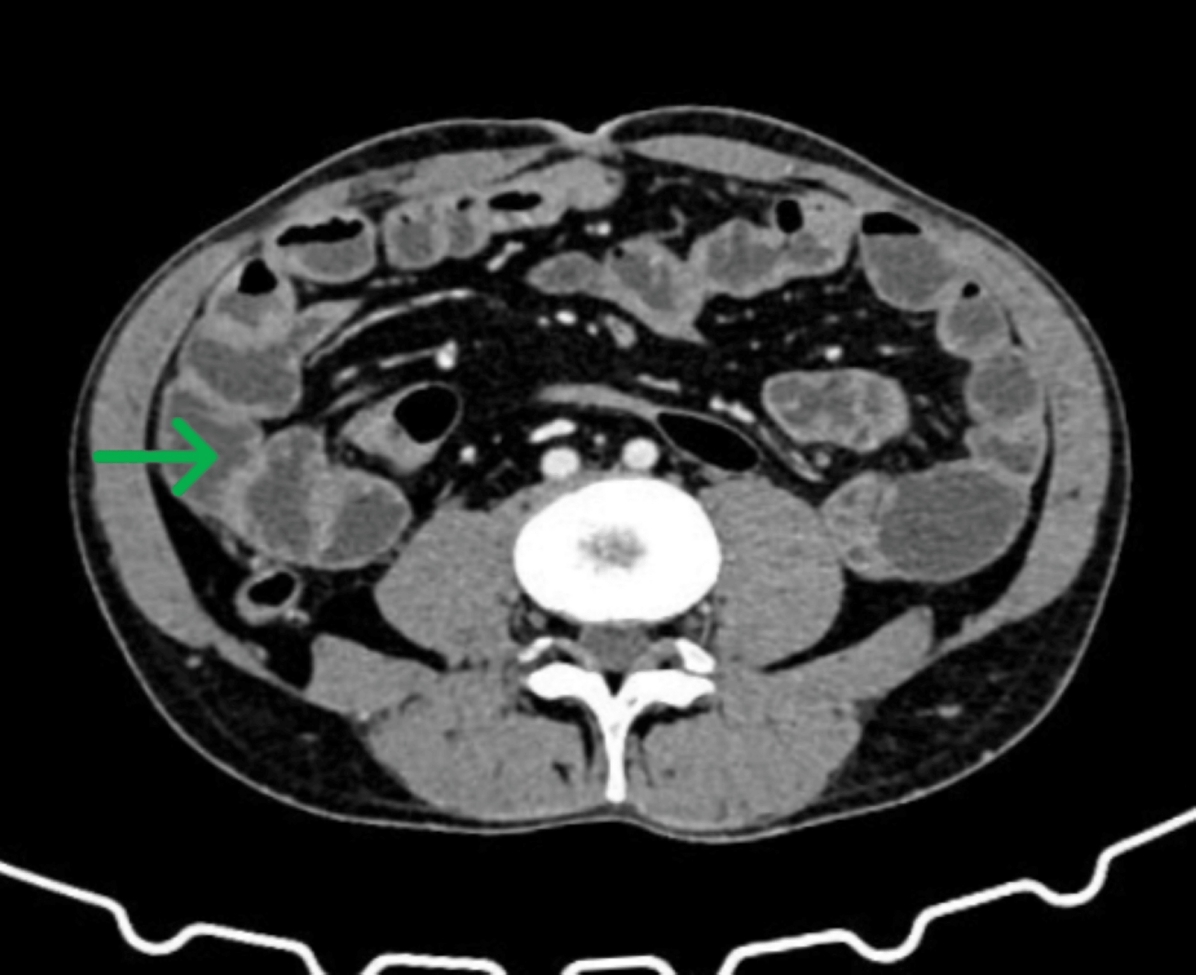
Triple-phase CT of the abdomen following one month of treatment with oral steroids. Findings compared to the previous study: No free fluid/collection was seen in the peritoneal cavity. The visualized bowel loops were not dilated.
